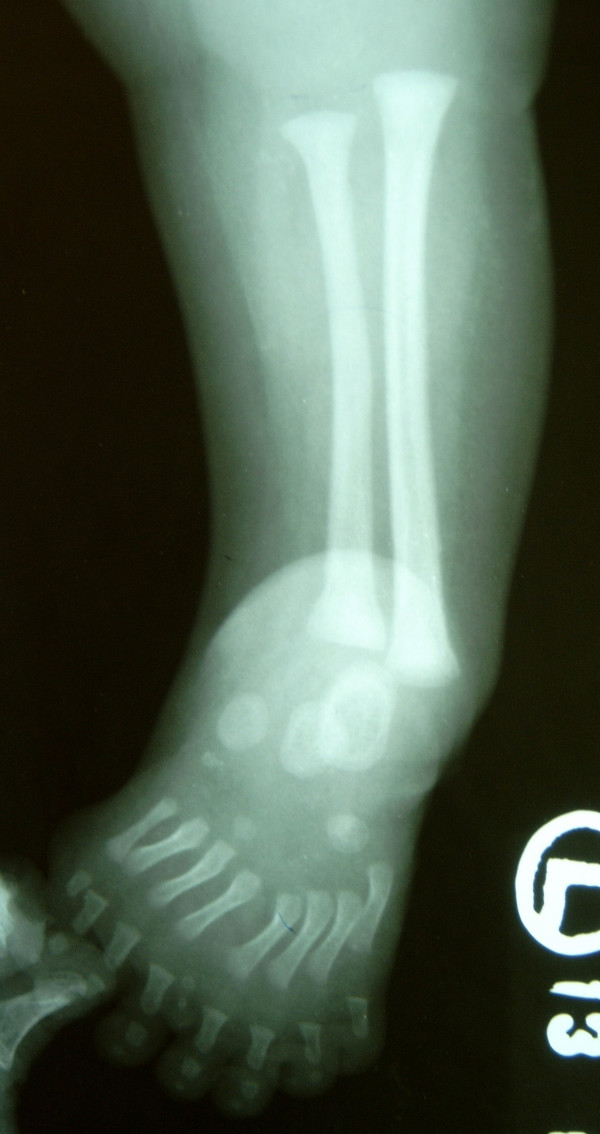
AP radiograph of the left lower limb with the foot showing two fibulae with multiple metatarsals.
